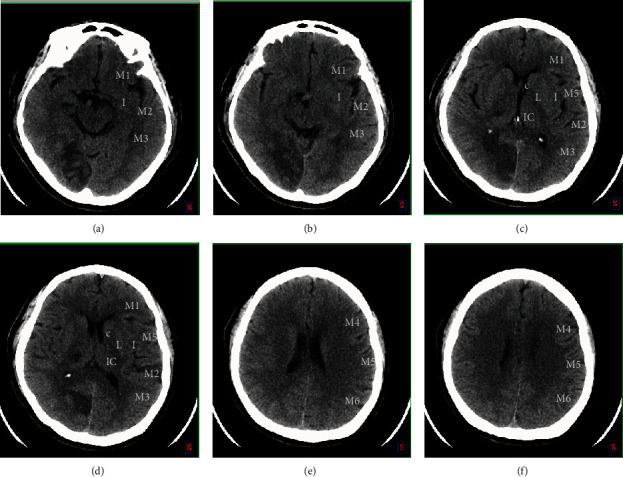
The MCA territory is divided into 10 ASPECTS regions, including caudate (C), insular ribbon (I), internal capsule (IC), lentiform nucleus (L), anterior inferior frontal cortex (M1), anterior temporal cortex lateral to the insular ribbon (M2), posterior temporal cortex (M3), anterior superior frontal cortex (M4), posterior frontal cortex (M5), and parietal cortex (M6). The M4, M5, and M6 are the MCA territories superior to the M1, M2, and M3 regions, respectively.
